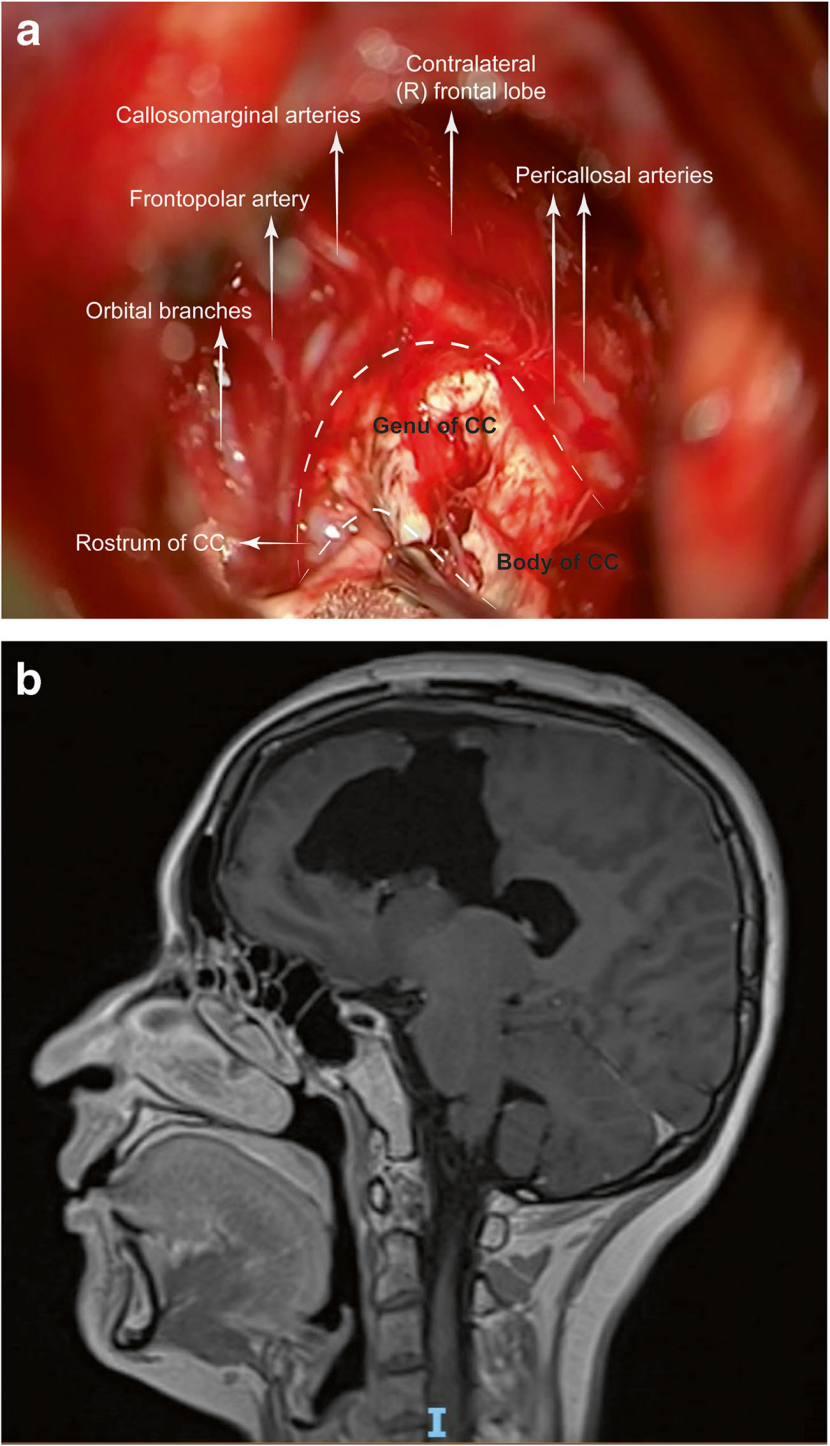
a The successful mapping and the combined subpial/interhemispheric dissection allowed clear anatomical visualization of critical structures and safe anatomo-surgical resection of the affected parts of the CC. b Sagittal post-operative MRI scan showing resection of the affected regions of the pre-SMA/SMA/cingulum and CC
